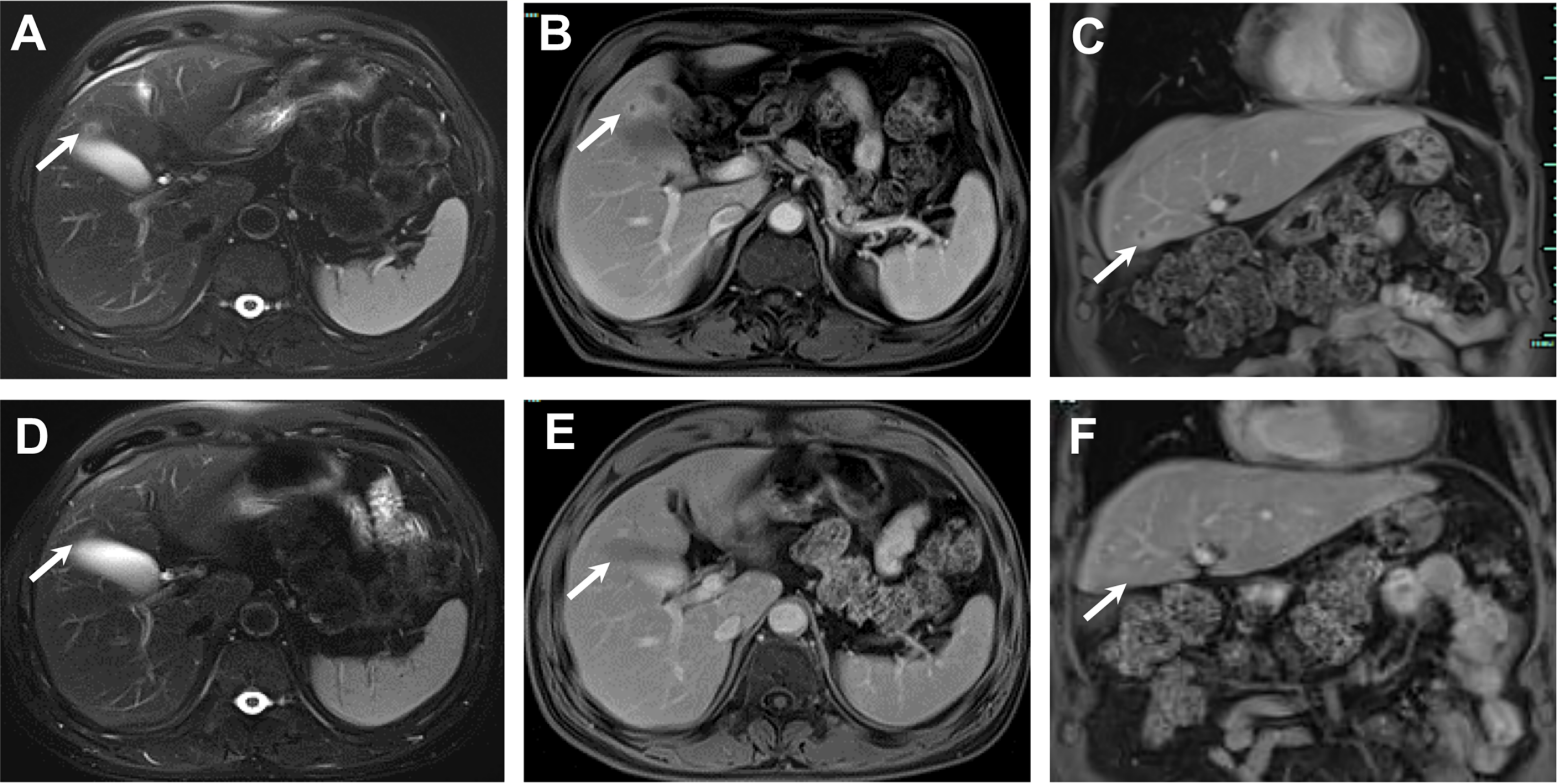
The MRI course of the SLR in liver. November 2020, 13 mm sized nodule in liver quadrate lobe showed circular hyperintensity on axial unenhanced T2-weighted image (A) and circular hyperenhancement in portal venous phase with axial image (B) and coronal image (C). January 2021, post 2 more doses of toripalimab, the nodule in liver quadrate lobe shrunk to 6 mm in diameter, axial unenhanced T2-weighted image (D) and axial enhanced T1-weighted image in portal venous phase with axial image (E) and coronal image (F).
